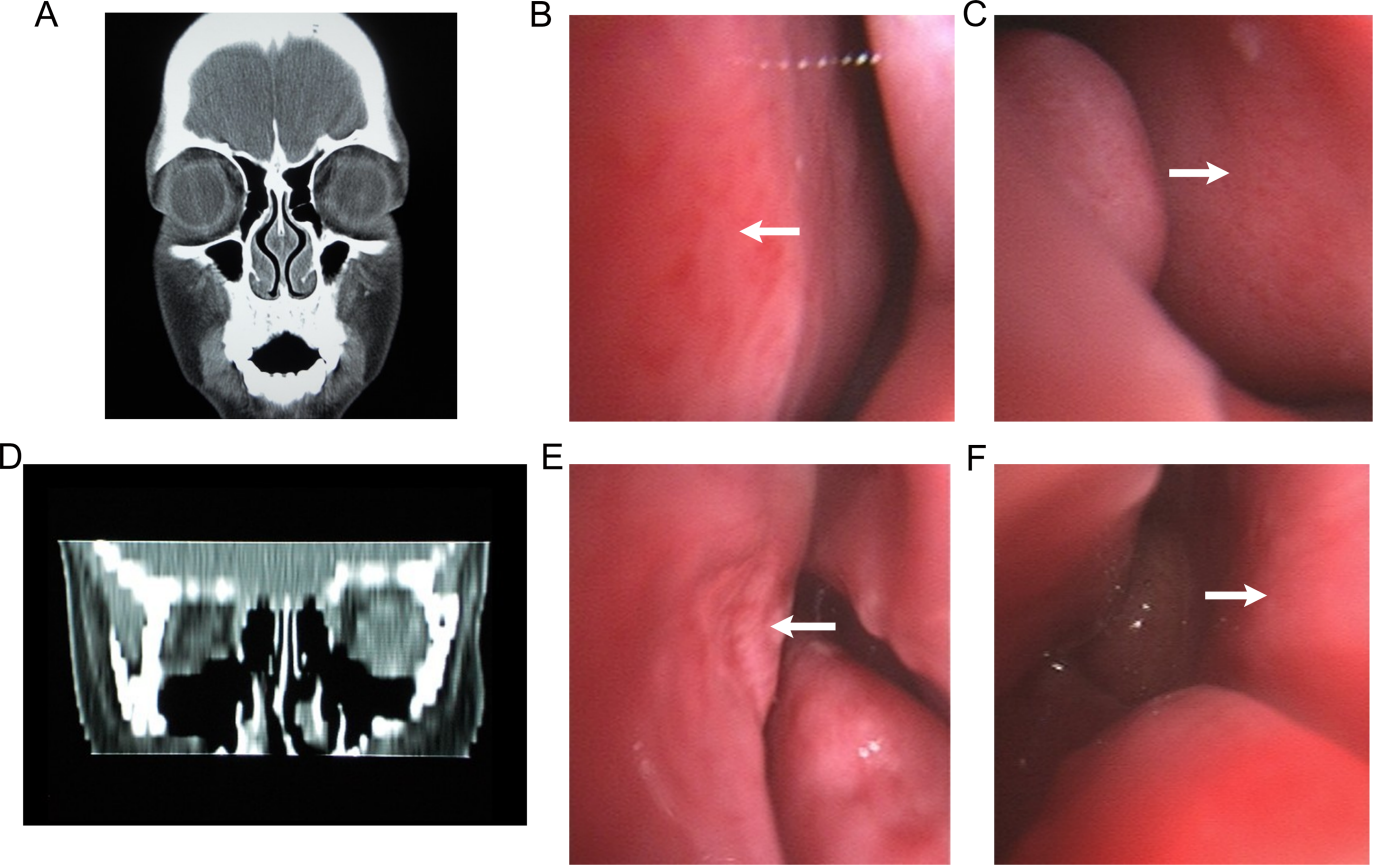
Results of paranasal sinus CT and electron-nasopharyngolaryngoscopy for typical cases with nasal stenosis. Electron-nasopharyngolaryngoscopy indicated the presence of bilateral nasal mucosal hyperemia and swelling, middle and inferior turbinate hypertrophy, as well as aperture-like stenosis of the bilateral nasal cavities.
